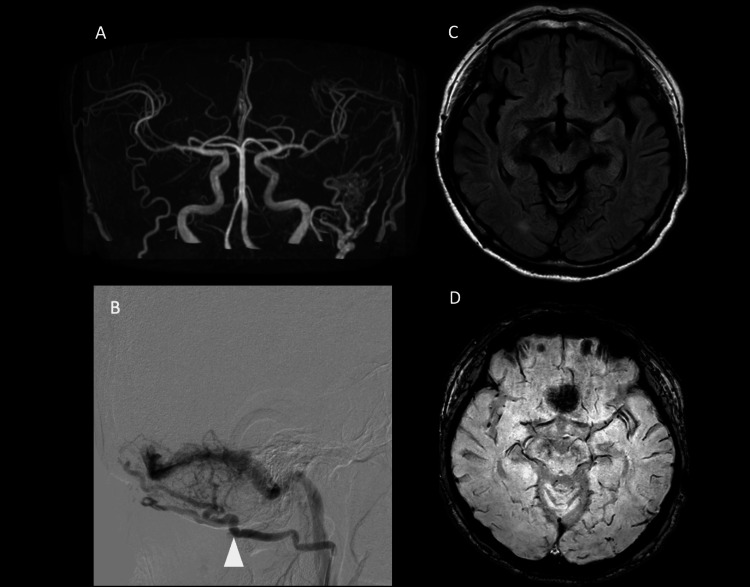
Initial MRI and cerebral angiography. MRA shows TS-SS dAVF (A). Cerebral angiography reveals a shunt formation at the transverse sinus primarily fed by the occipital artery (white arrowhead) without CVR (B: lateral view) (Cognard IIa). No FLAIR hyperintense lesions can be observed (C). SWI showed no venous dilation (D). MRI: magnetic resonance imaging; MRA: magnetic resonance angiography; TS-SS: transverse sinus-sigmoid sinus; dAVF: dural arteriovenous fistula; CVR: cortical venous reflex; FLAIR: fluid-attenuated inversion recovery; SWI: susceptibility-weighted imaging
